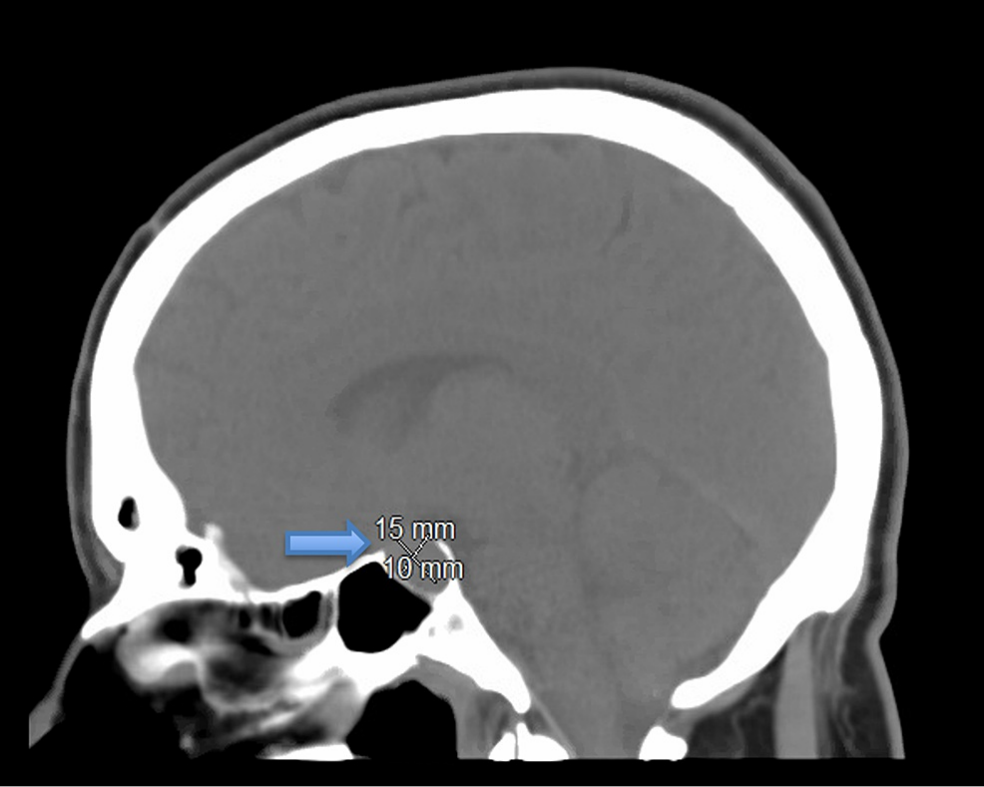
CT of the head without contrast: sagittal view demonstrating prominence of the pituitary gland measuring 1.0 × 1.5 cm with ill-defined hyper-attenuation along the superior aspect. CT: computed tomography
